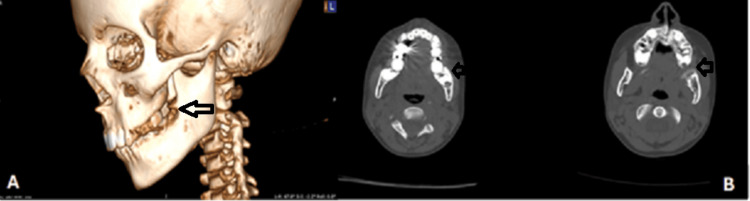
Preoperative CT scan of the patient. (A) Three-dimensional reconstruction showing the lesion adjacent to tooth #37. (B) Axial sections showing discontinuity of the buccal cortex at the left side of the mandible.
